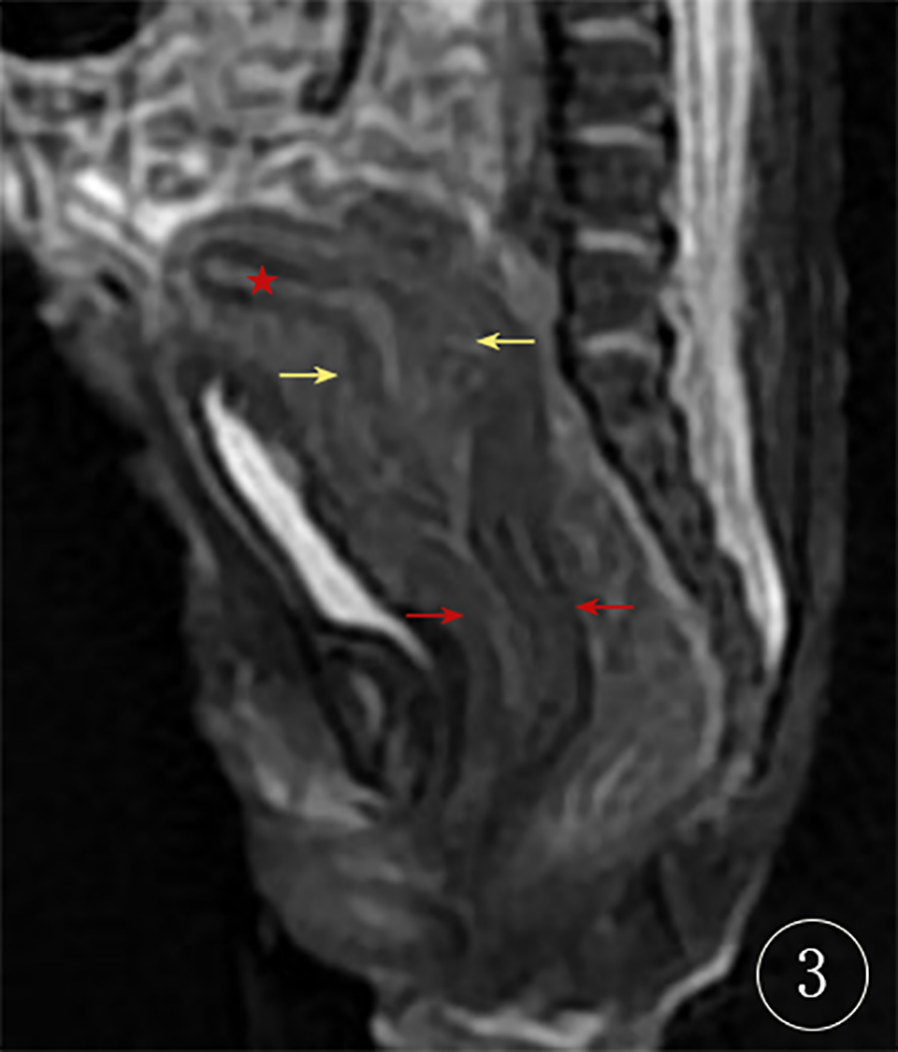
Neonatal MRI at 1 day postpartum the red star (★) represents the uterine cavity, the yellow arrow indicates the cervix, and the red arrow points to the thickened vaginal wall.
